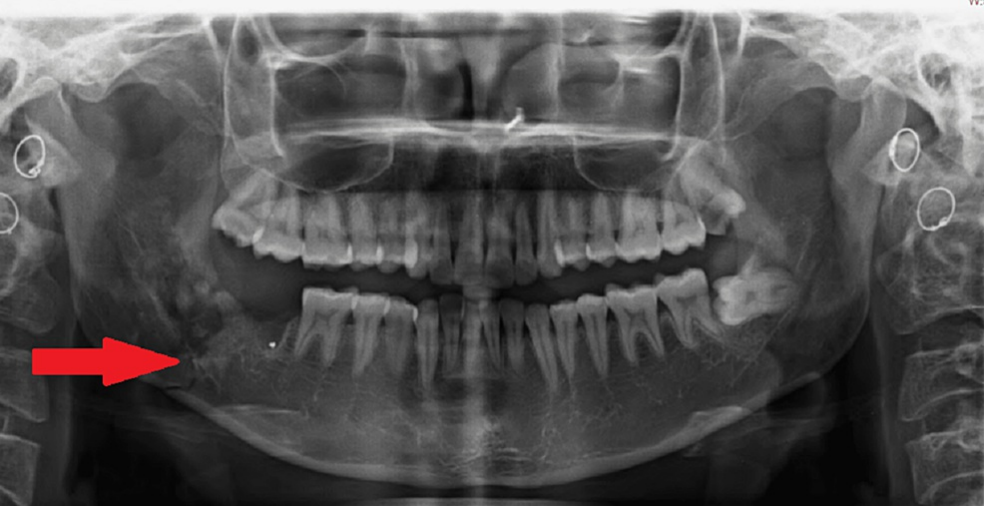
Orthopantomogram showing oblique radiolucent line extending from the extraction socket of the right third molar fracturing the right angle of the mandible (arrow).
